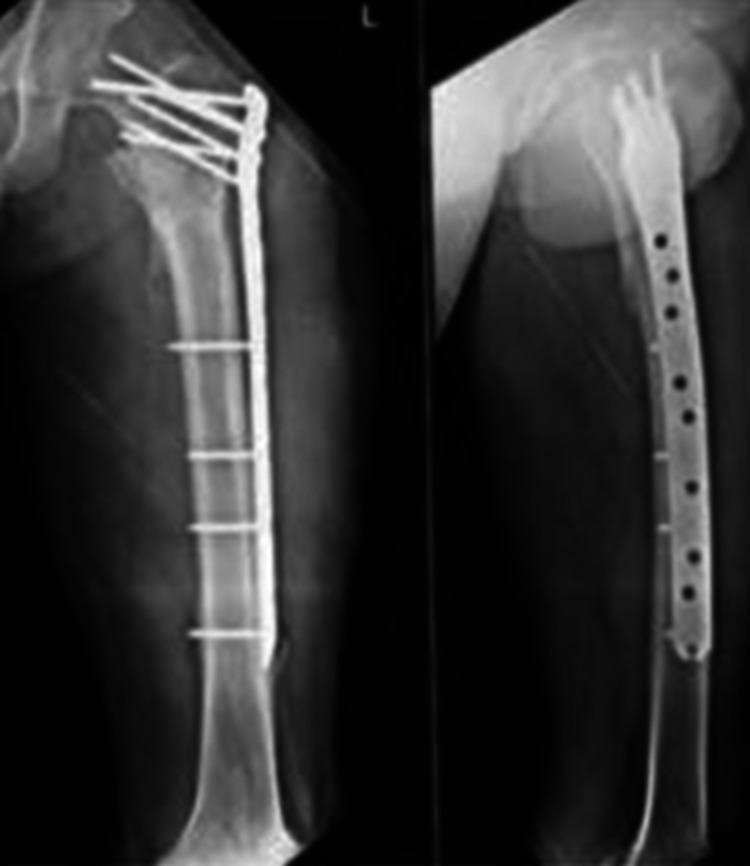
Antero-posterior and lateral radiographs showing union at the fracture site at six months of follow up
